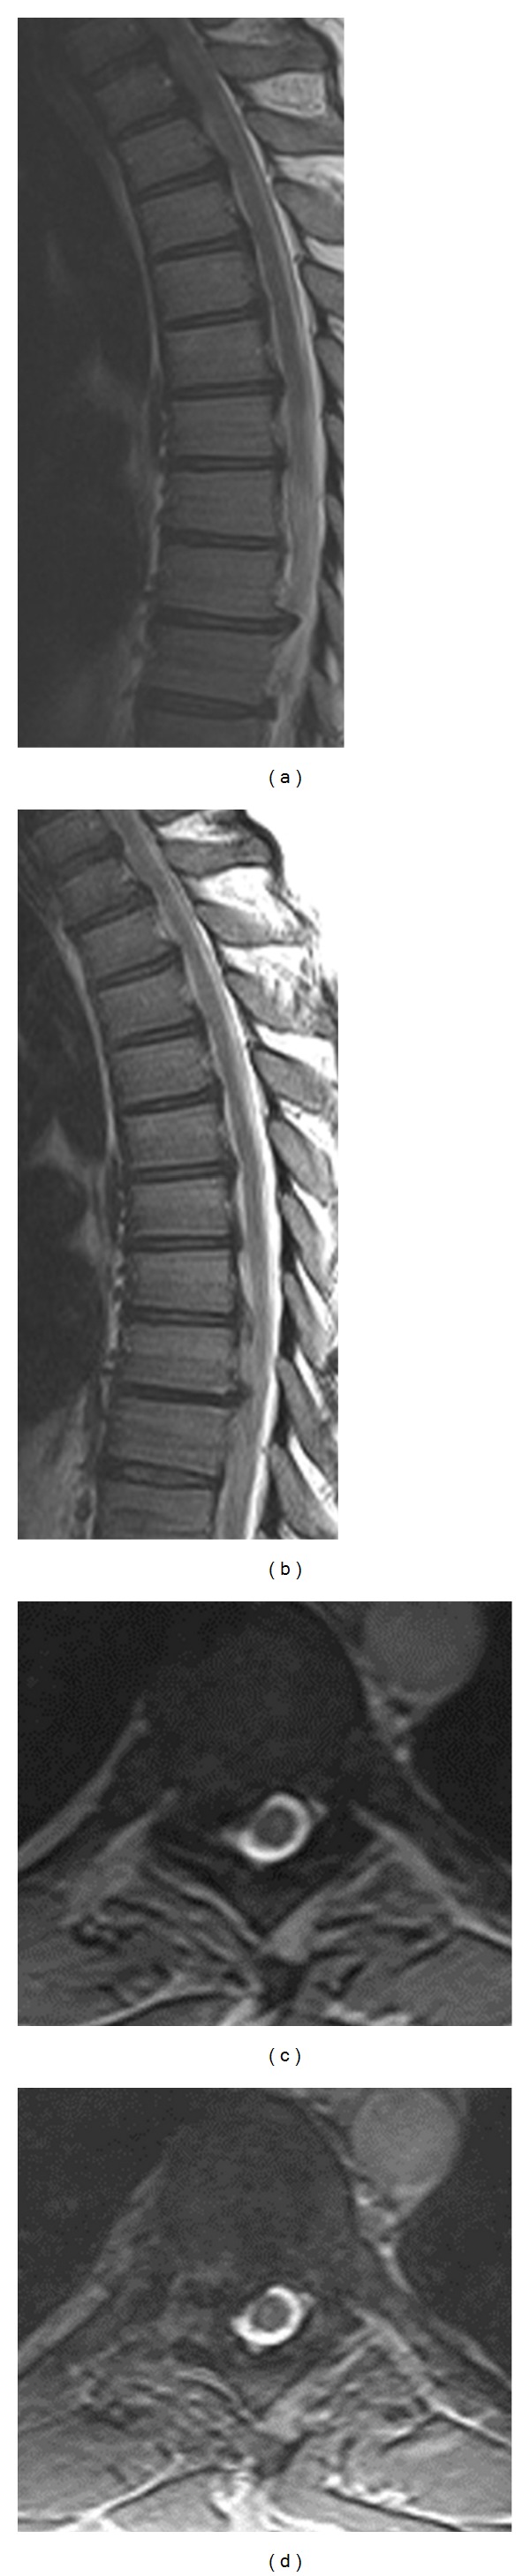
Preoperative MRI images of a T8-9 disc herniation compressing the spinal cord, which caused the patient to have mid back pain radiating to the shoulder blade ((a) and (b)). Postoperative MRI images showing removal of the extruded disc material ((c) and (d)).
